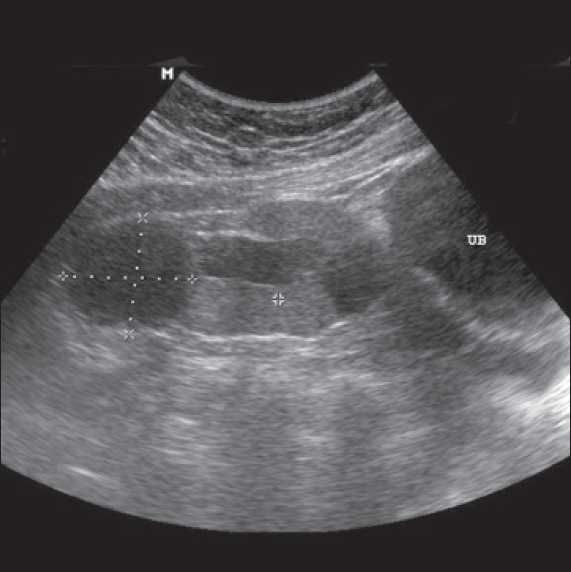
Ultrasound showing right Pelvic Kidney
